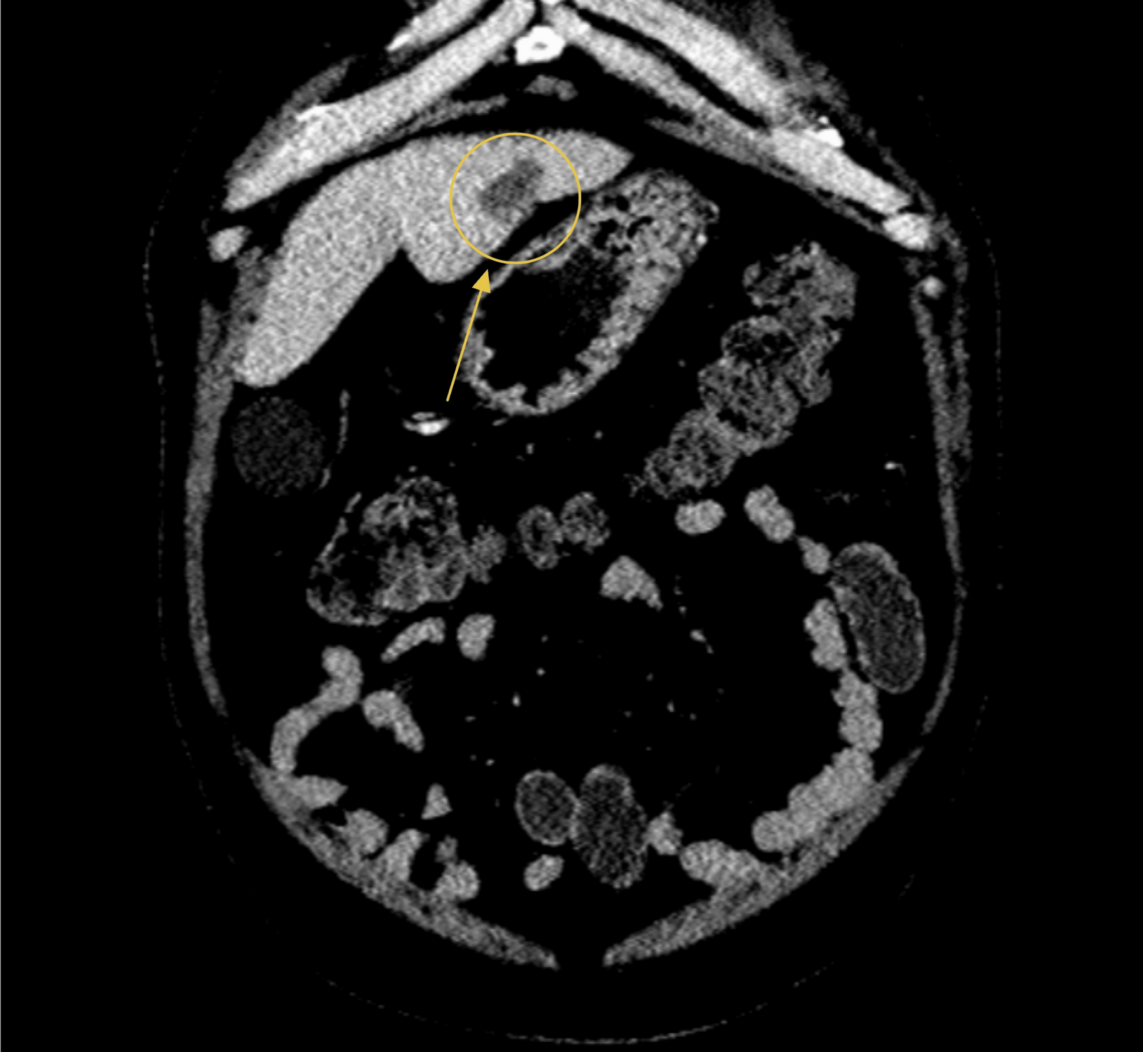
Hypodense hepatic lesion in the anterior portion of the left hepatic lobe on CT
